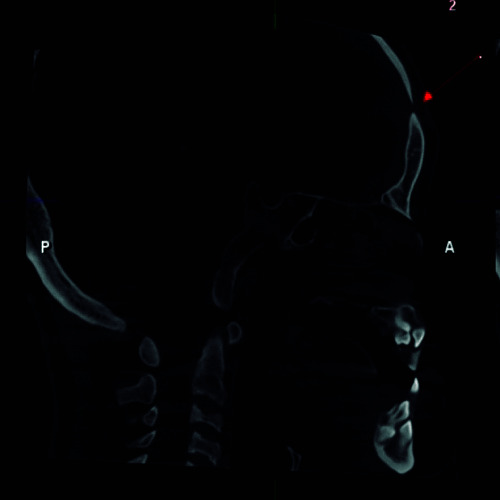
Sagittal section of CBCT of a patient with CCD showing the lack of closure of the frontal suture.
